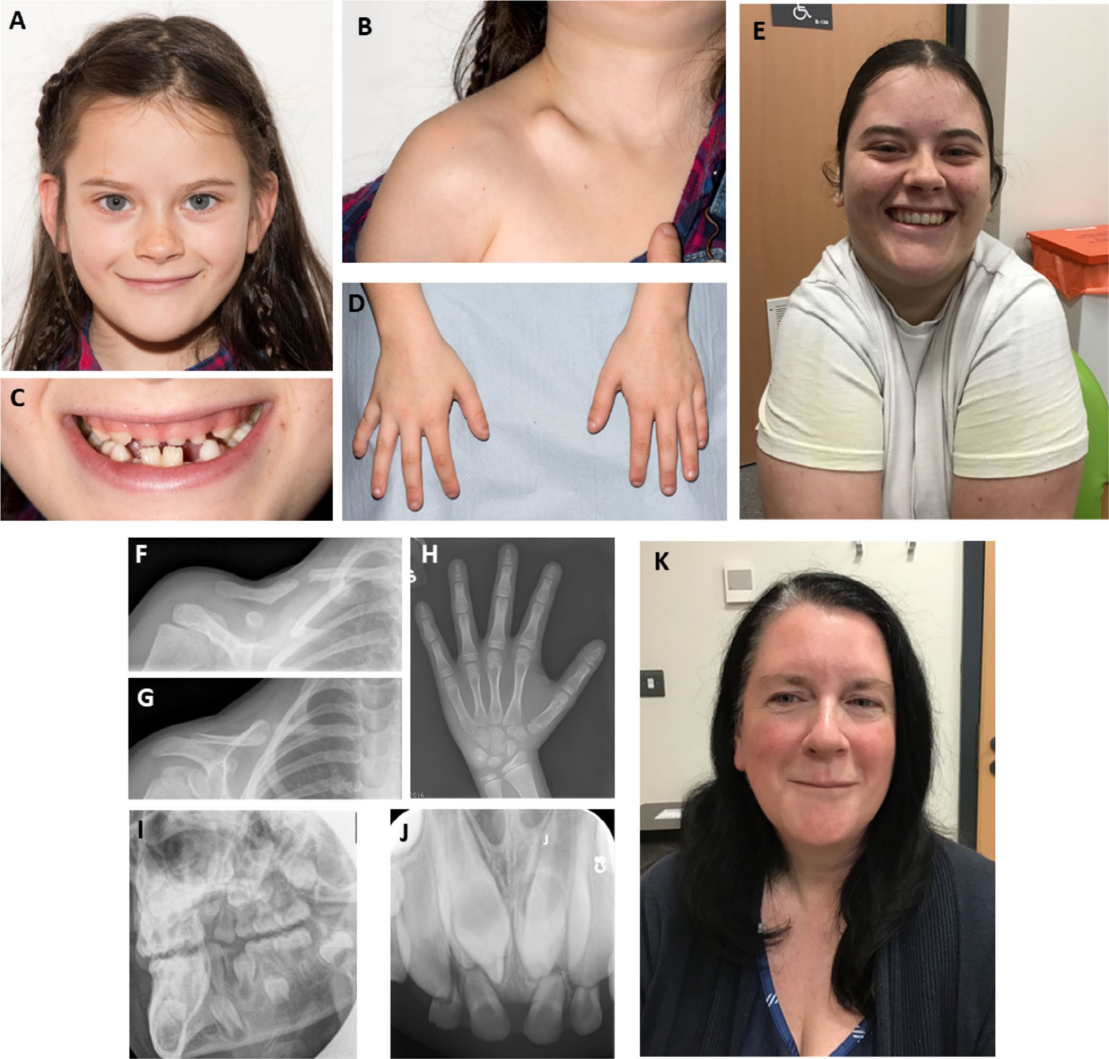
Images for family with CCD. (A) Proband at 9y without a marked facial difference, (B) right clavicular prominence at site of pseudoarthrosis, (C) irregular dentition, (D) short digits. (E) Marked shoulder approximation at 16y. (F,G) Anteroposterior and oblique radiographs of right clavicle at 5y showing mid‐clavicular pseudoarthrosis. (H) Anteroposterior radiograph of left hand at 8y shows a pseudoepiphysis of first metacarpal, hypoplastic terminal phalanges and acro‐osteolysis of terminal phalanges. (I,J) Lateral oblique of mandible and AP dental radiograph at 8y show supernumerary teeth. (K) Proband's mother also without facial signs of CCD.
